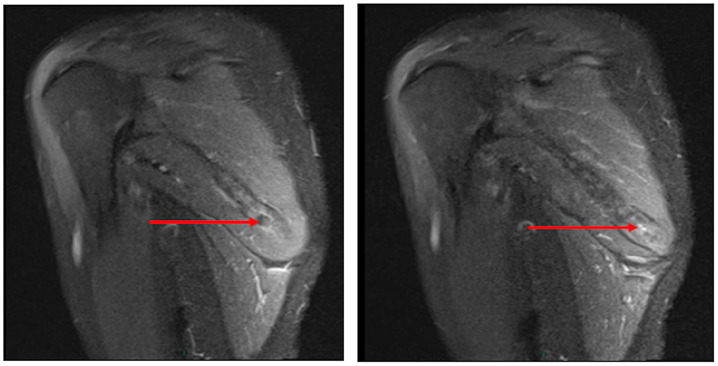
T2-weighted MRI images of right scapula. Standard sequences without contrast sagittal T2-weighted images of right scapula. Images confirm soft tissue oedema within the serratus anterior with residual bone marrow oedema within the inferior angle of the scapula in keeping with tendinopathy/enthesopathy (red arrows).
